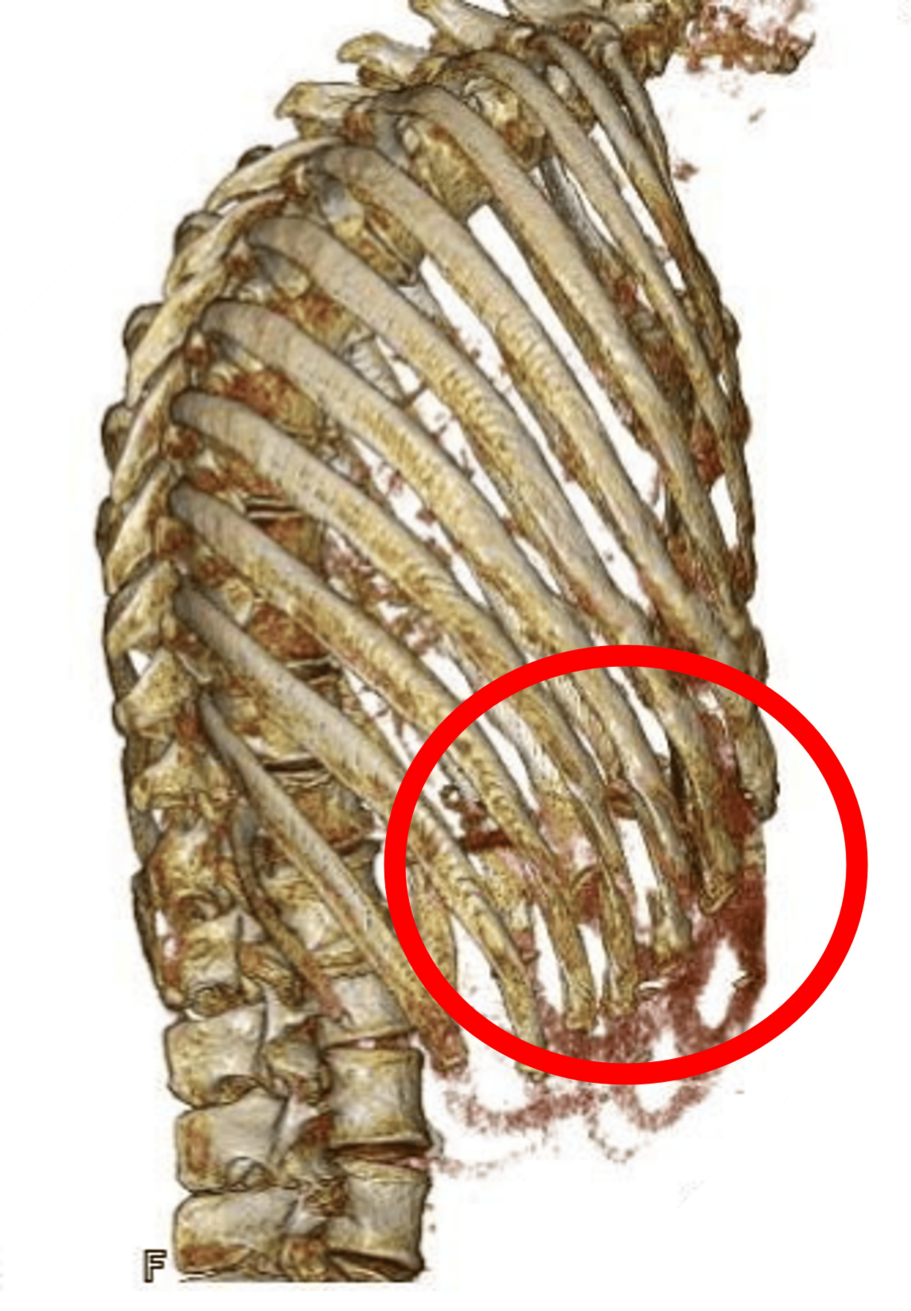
Preoperative three-dimensional chest computed tomography. The red circle indicates multiple rib fractures on the right at the level of the seventh to ninth thoracic vertebrae
